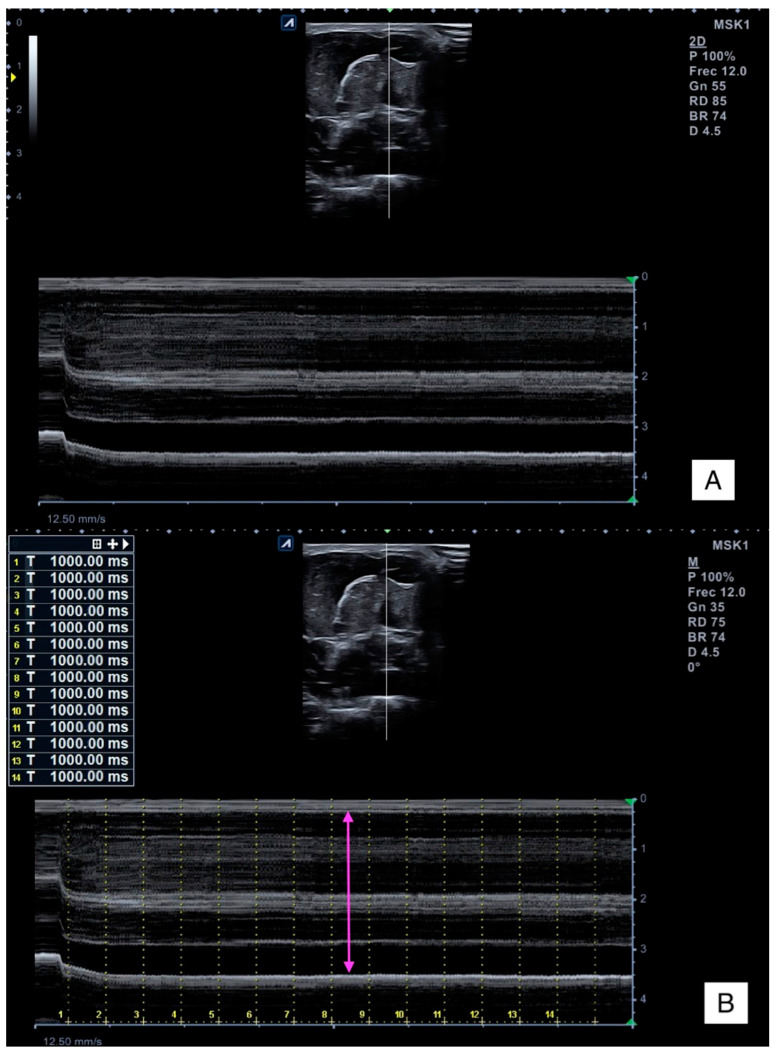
Raw M-mode ultrasound imaging (A) and thickness measurement (B).
